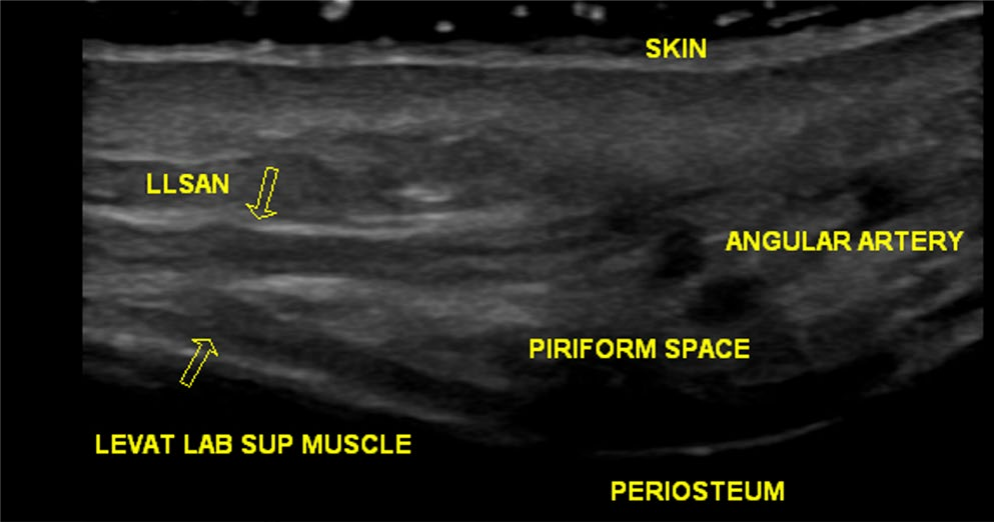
High‐frequency ultrasound image of the same patient's medial infraorbital region, showing the underlying muscular anatomy, including the LLSAN.
